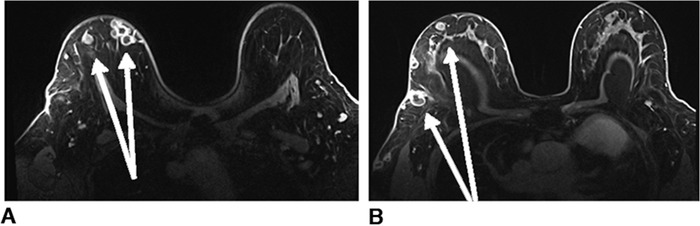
Bilateral breast magnetic resonance imaging shows (A) multiple breast abscesses (arrows) in the right breast and (B) a normal (arrows) left breast.
